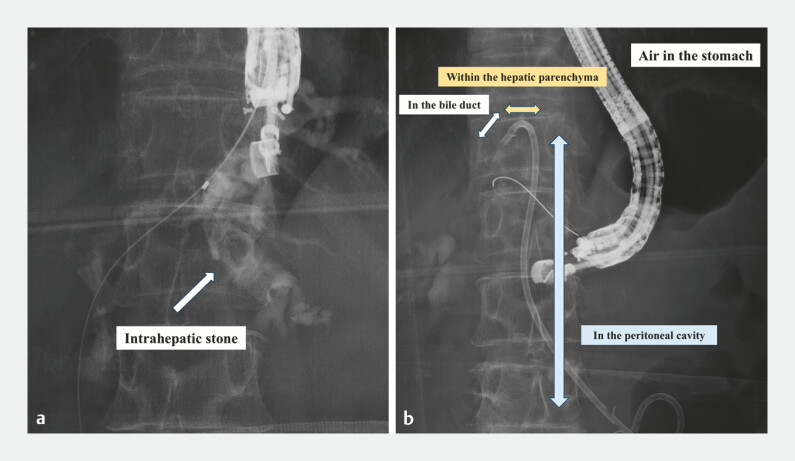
A fluoroscopic image of endoscopic ultrasound-guided hepaticogastrostomy.
